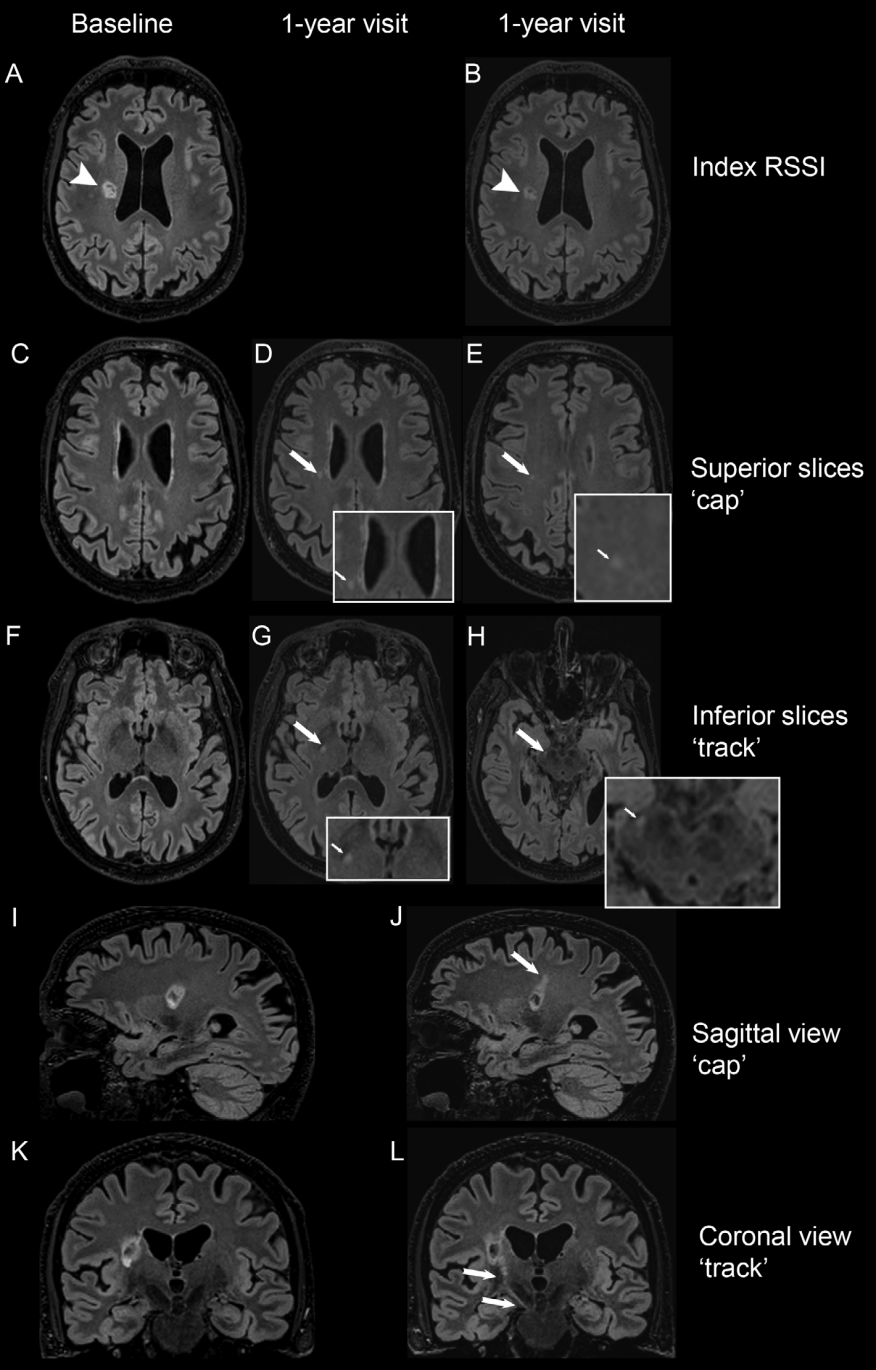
Example of ‘caps’ and ‘tracks’ following recent small subcortical infarct (RSSI) at the infero‐superior direction. The first column represents the baseline FLAIR MRI images performed 78 days after stroke onset, the second and third columns represent the 1‐year follow‐up FLAIR MRI images performed 476 days after stroke onset. (A, B) The index RSSI (arrowheads) is visible in the right centrum semiovale on baseline and 1‐year MRI. (C–E) On the MRI slice superior to the index RSSI, there is no abnormality at baseline. At 1 year, new ‘caps’ appear as hyperintensities in 2 axial slices superior to the index RSSI (arrows and augmented squares). (I, J) In the sagittal plane, the ‘cap’ is visible as a trajectory ascending from the index RSSI (arrow). (F–H) On the MRI slice inferior to the index RSSI, there is no abnormality at baseline. At 1 year, new ‘tracks’ appear as hyperintensities in 2 axial slices inferior to the index RSSI (arrows and augmented squares). (K, L) In the coronal plane, the ‘track’ is visible as a trajectory descending from the index RSSI toward the internal capsule and cerebral peduncle, following the corticospinal tract (arrows).
